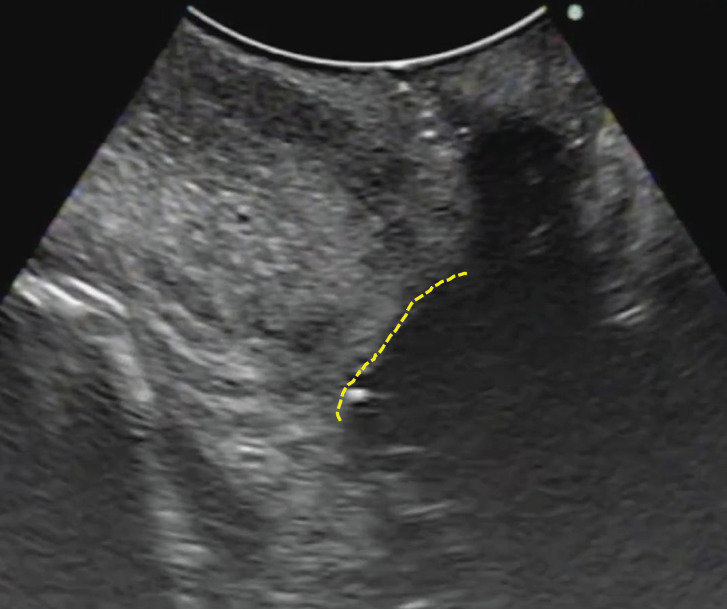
Intraoperative transoral ultrasonography (TOUS) image following en bloc resection TOUS image following en bloc resection of the cyst, tract, and central portion of the hyoid bone. The dotted line indicates the tongue-side dissection plane. No residual cystic structure is observed, confirming complete excision.
